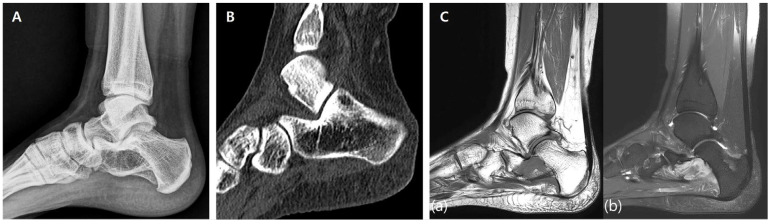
Preoperative imaging. (A) Preoperative X-ray showing a well-demarcated lesion within the calcaneus with cortical thinning and intact surrounding bone structures. (B) Preoperative CT scan demonstrating a 25 × 12 × 15 mm hypodense lesion in the calcaneal body, with thinning of the cortical bone and no evidence of periosteal reaction. (C) Preoperative MRI. (a) T1-weighted image showing homogenous low signal intensity. (b) T2-weighted image showing heterogeneous high signal intensity with central low-signal areas.
